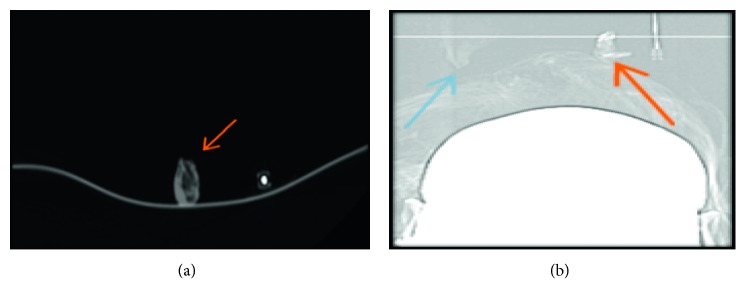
Axial CT scan (a) and scout (b) of gauzes removed from both right (red arrow) and left (blue arrow) nostrils, after being inserted intentionally by the author (C.I.) during real-time cisternogram. This technique confirmed without doubt the presence of leaky contrast in the right nostril, compatible with CSF leakage.
